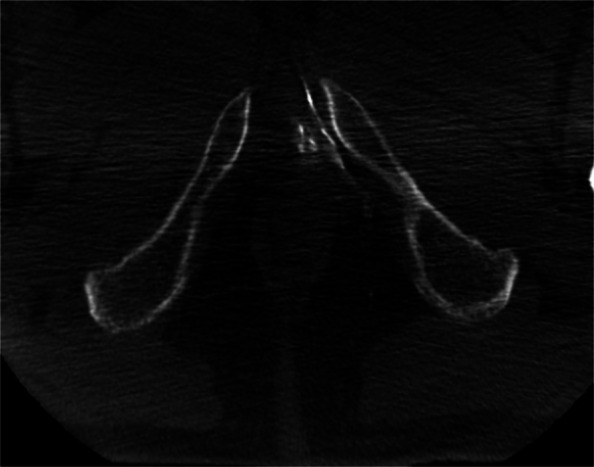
CBCT demonstrating non-target penile enhancement
